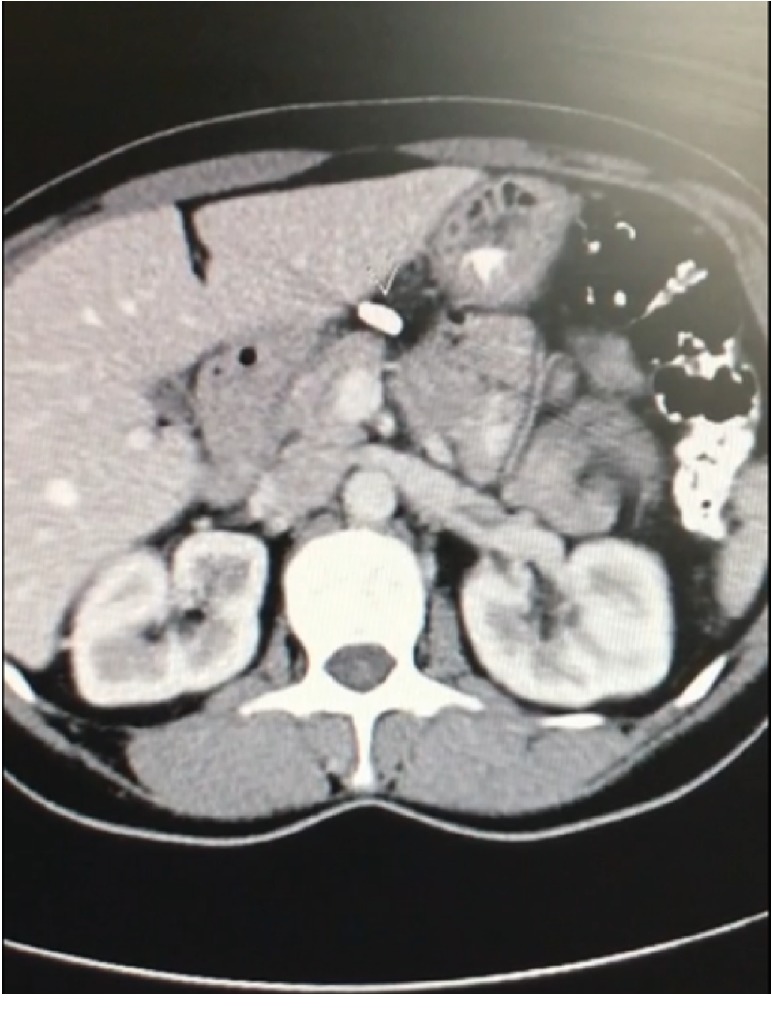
Transverse section of a computed tomography scan of the abdomen showing a migrated Filshie clip lying inferior to the left of the liver.
